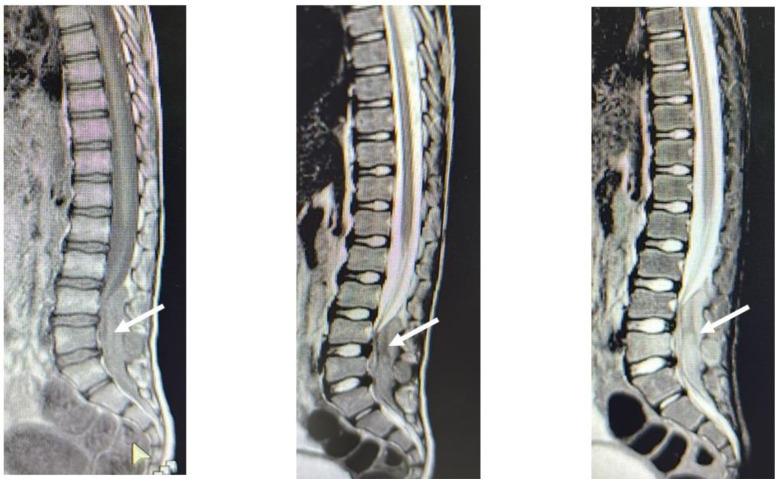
Case 2: MRI lumbo-sacral spine: A 7.8 x 1.6 cm enhancing soft tissue intra-spinal mass from the upper border of L4 up to S2 level with extension through neural foramina into para-spinal regions.
